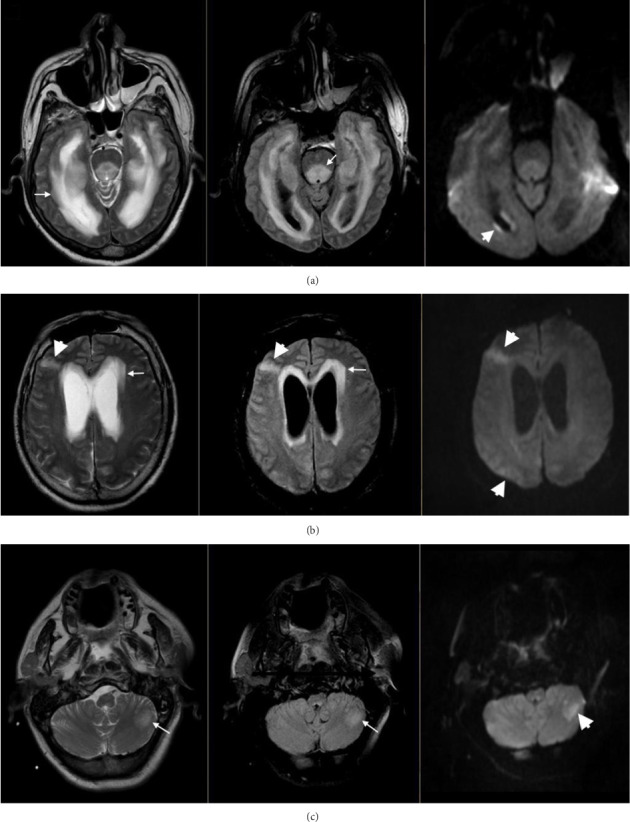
MRI findings of nosocomial neurolisteriosis in a 61-year old man with non-Hodgkin's lymphoma on chronic corticosteroid therapy. On Day 45 of hospital stay for cancer treatment, he presented with meningoencephalic symptoms. MRI was performed on Day 3 after neurological symptoms onset. From left to right, axial T2-weighted, FLAIR, and DWI sequences. (a) Diffuse hyperintense signal of the periventricular white matter and the periaqueductal region of the mesencephalon (arrows) and focal lesions of the right side periventricular white matter with increased DWI evocative of acute ischemic images (arrow head). (b) Hydrocephalus with periventricular transependymal edema (arrows), and focal lesions with hyperintense signal of the right frontal and parietal cortical matter on T2-weighted and FLAIR sequences with increased DWI evocative of acute ischemic images (arrow heads). (c). Focal left cerebellar lesion hyperintense on T2-weighted and FLAIR sequences (arrow) with increased DWI evocative of acute ischemic image (arrow head). FLAIR = flow attenuated inversion recovery. DWI = diffusion weighted imaging.
